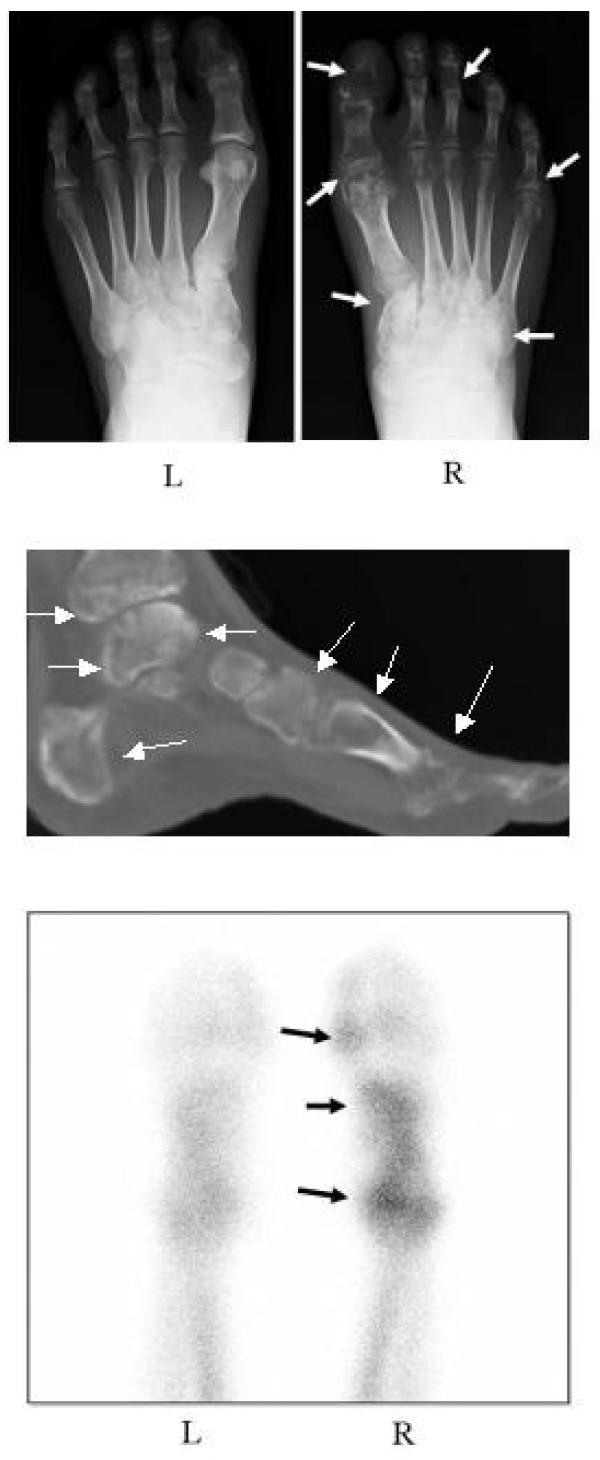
Radiograph (A), reconstitution computed tomography (CT) image (B), and scintigraph (C) of the both feet before treatment. The radiograph shows regional osteoporotic changes in the phalanges, metatarsals, and tarsals of the right foot compared with the normal, left foot (white arrow) (A). Reconstitution CT image exhibits the extensive osteoporotic changes in the foot and ankle (white arrow) (B). Bone scintigraphy with ^99 m^Tc-methylene diphosphonate shows a marked increase in radioactivity in the right foot (black arrow) (C).
